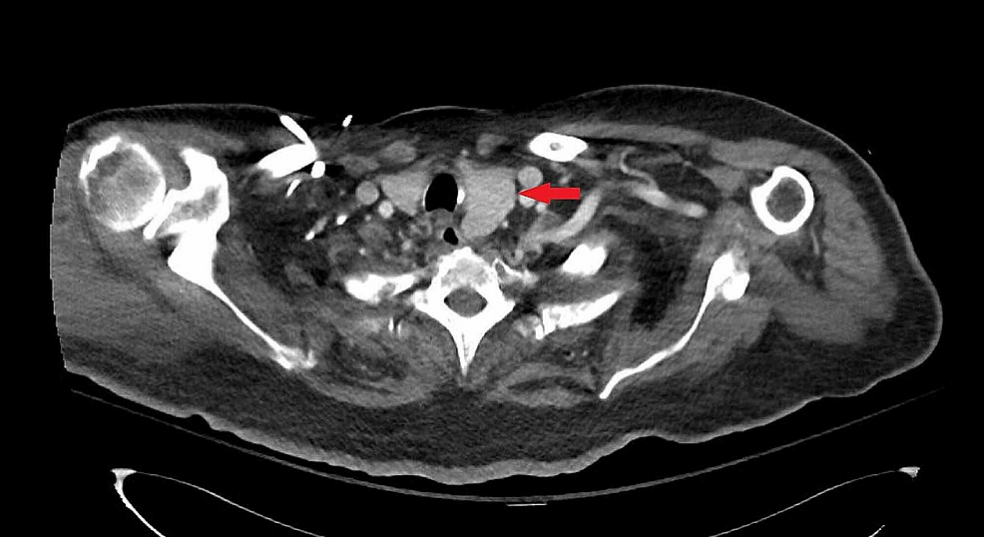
NCCT of the soft tissues of the neck showing prominent left thyroid lobe. NCCT = non-contrast computed tomography
